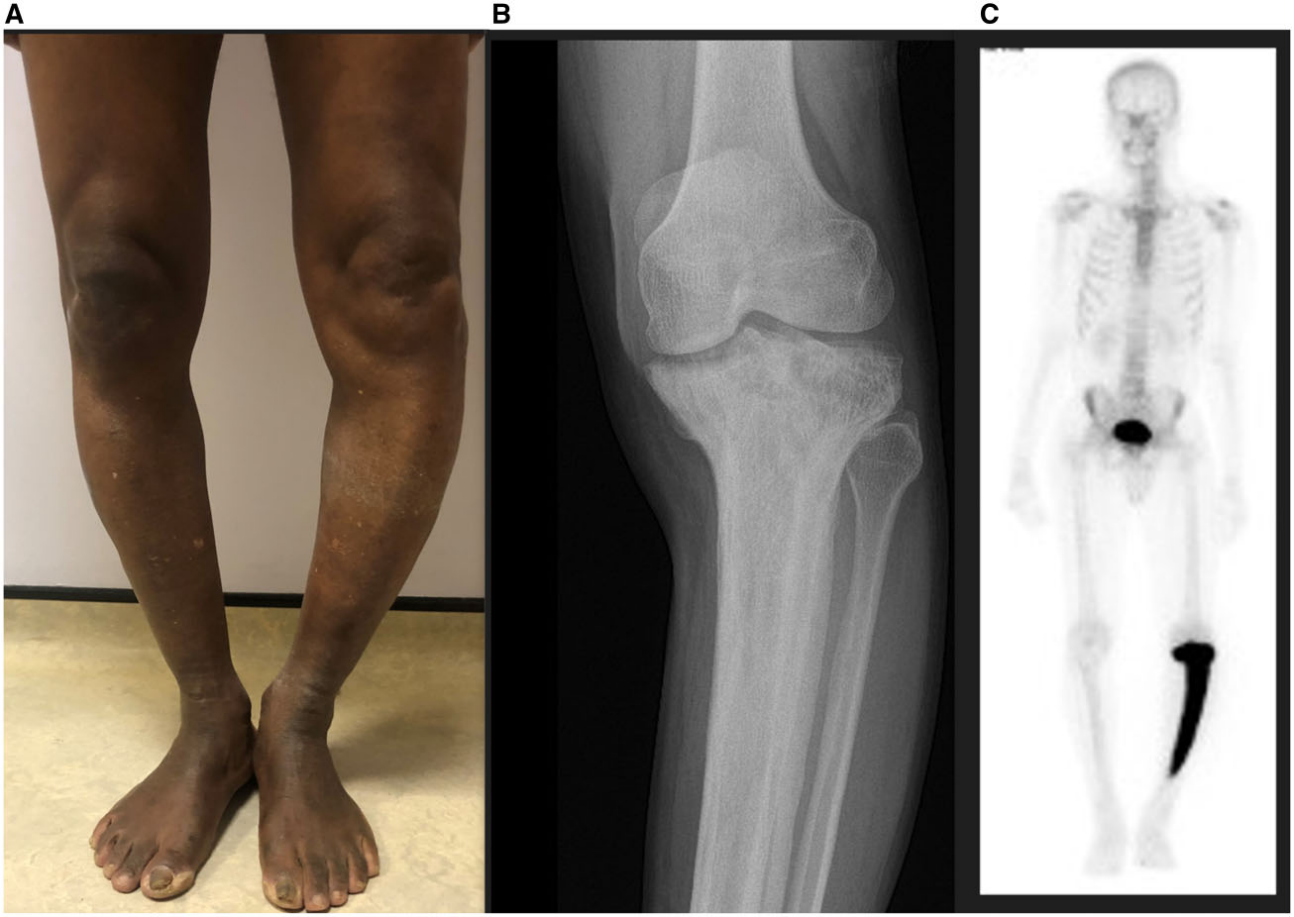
Clinical images and radiographic depiction of sabre tibia in Paget’s disease. (A) Prominent sabre-like convexity of the right leg. (B) Radiograph (anteroposterior view) showin cortical thickening, trabecular enlargement and bowing of the tibia. (C) SPECT CT showed increased activity in the proximal two-thirds of the tibia
